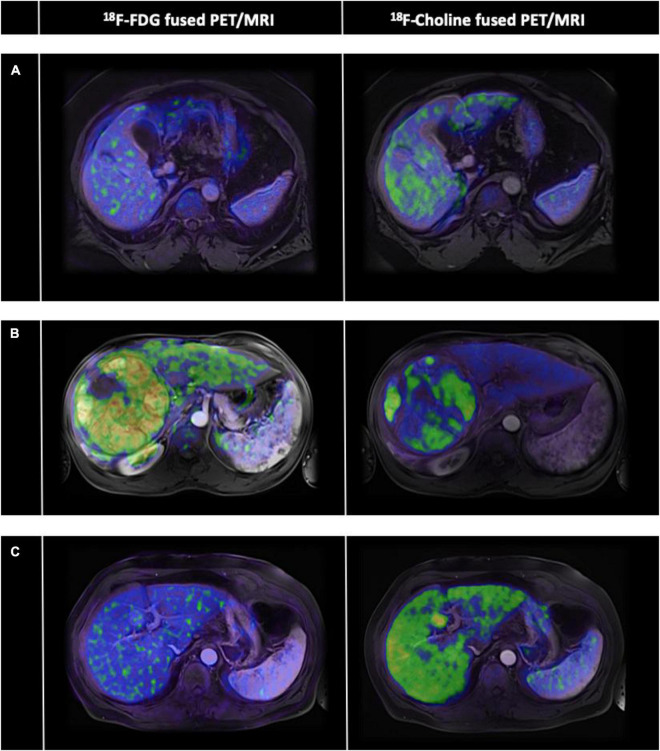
Case-mix of hepatocellular carcinoma (HCC) with various dual-tracer positron emission tomography (PET) profiles. The PET part of the non-contrast-enhanced PET/computed tomography (CT) data 18F fluorodeoxyglucose (18F-FDG on the left panel and 18F-choline on the right panel) of three HCCs (A–C) were retrospectively fused to their available contrast-enhanced MRI data (axial T1-weighted MRI pulse sequences with arterial phase contrast enhancement). (A) Dual-PET radiotracers negative uptake (HCC Grade III, with mVI). (B) Dual-PET radiotracers positive uptake (HCC Grade I, with mVI). (C) Negative 18F-FDG uptake but positive 18F-choline uptake (HCC Grade I-II, without mVI).
